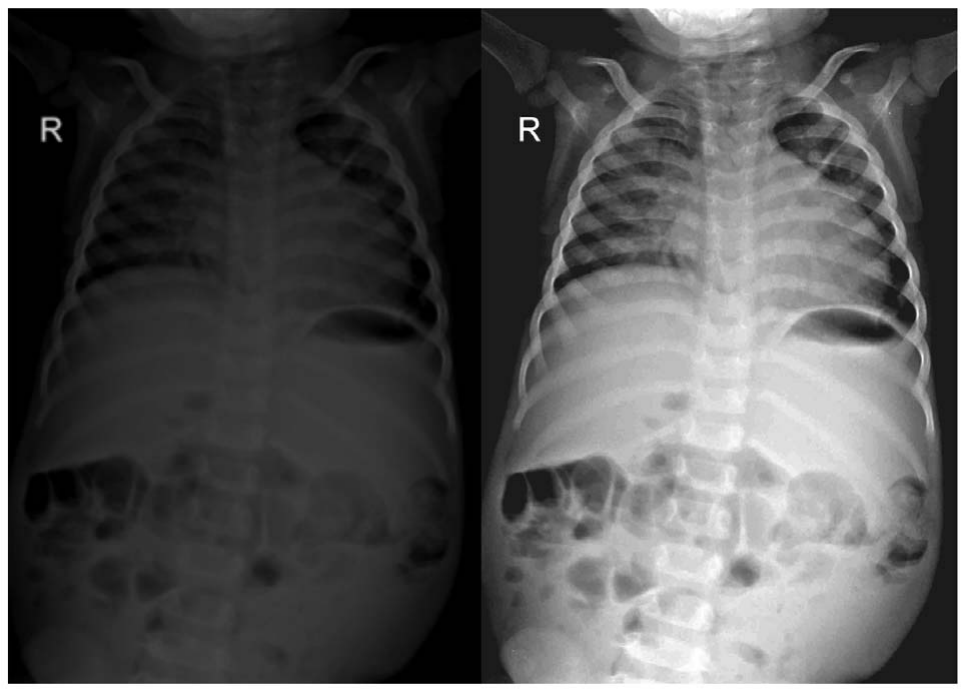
Post processing functions with Digital X- Rays. Note: This is an example of how the quality of digital X-rays could be enhanced by image processing. Depicted on the left is the original X-ray, on the right the same X-ray after digital image manipulation.
